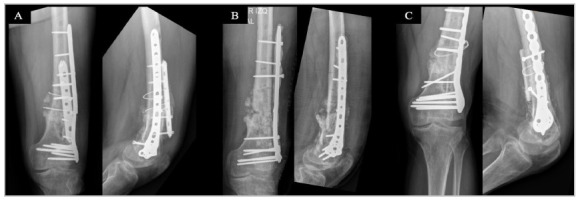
Case 2. A. Antero-posterior (AP) and lateral radiographs of the distal region of the left femur, showing osteosynthesis failure, with plaque breakage and oligotrophic infected pseudarthrosis of the distal femur metaphysis; B. The implants were removed and an antibiotic cement-coated block plate was used for stabilization. AP and lateral radiographs show persistent nonunion; C. The second reconstructive surgery was performed, with implant removal and bone healing with a structural allograft. AP and lateral radiographs of the second reconstructive surgery showing the new fixation and structural graft.
